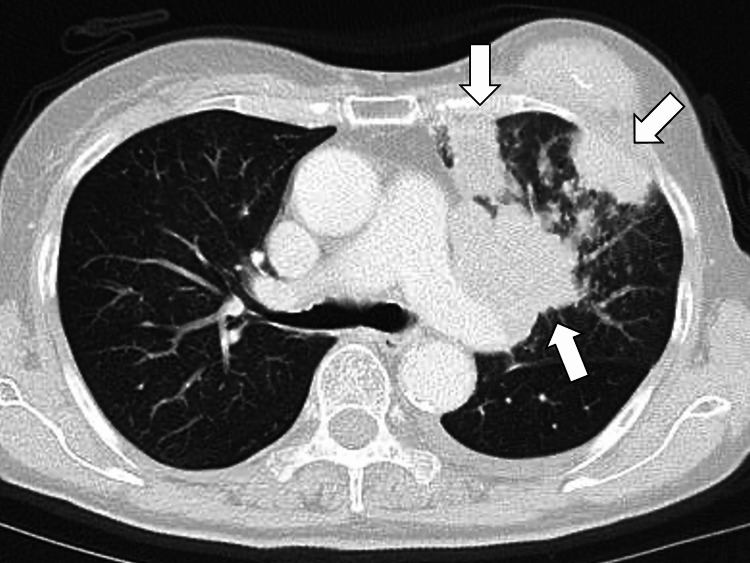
Chest computed tomography revealing multiple masses in the left upper lobe of the lung, associated with left hilar and mediastinal lymphadenopathy (white arrows).
